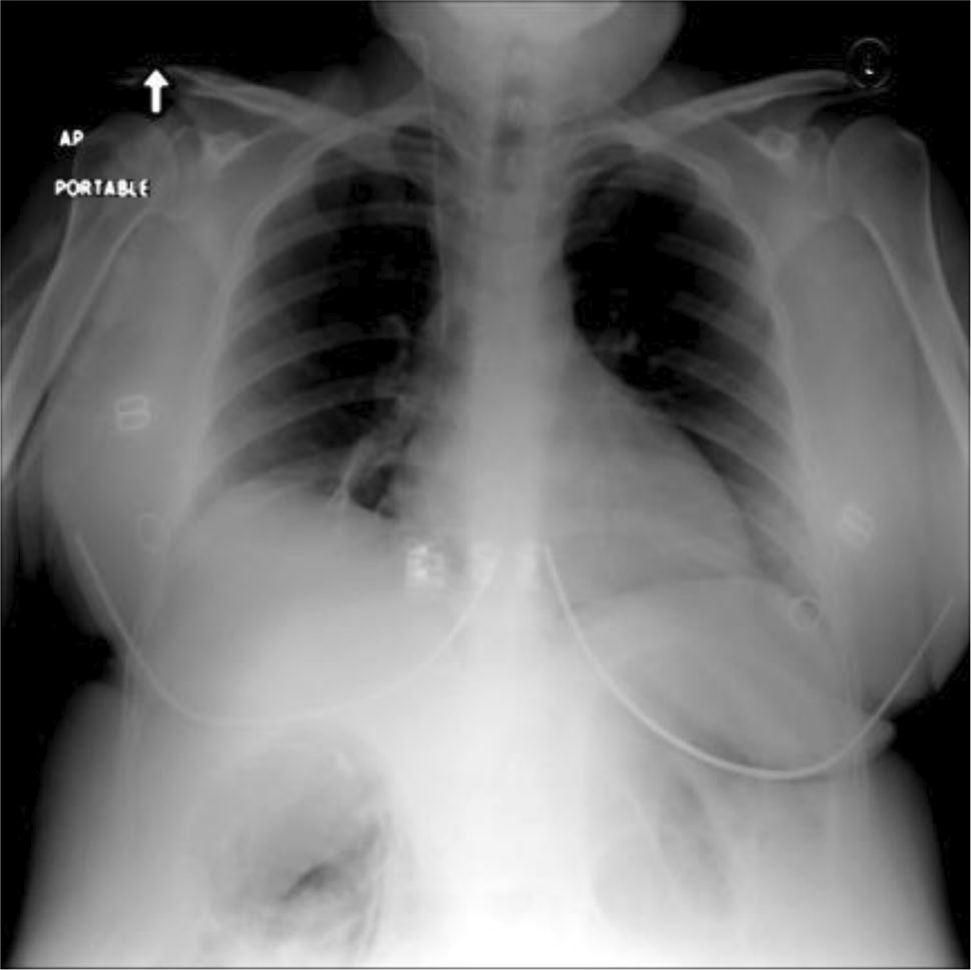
Example of a chest radiograph with the corresponding label 01110, where ‘0’ means no such lesion and ‘1’ means the presence of such lesion, indicating that this chest radiograph contains three types of lesions: Cardiomegaly, Effusion, and Consolidation.
